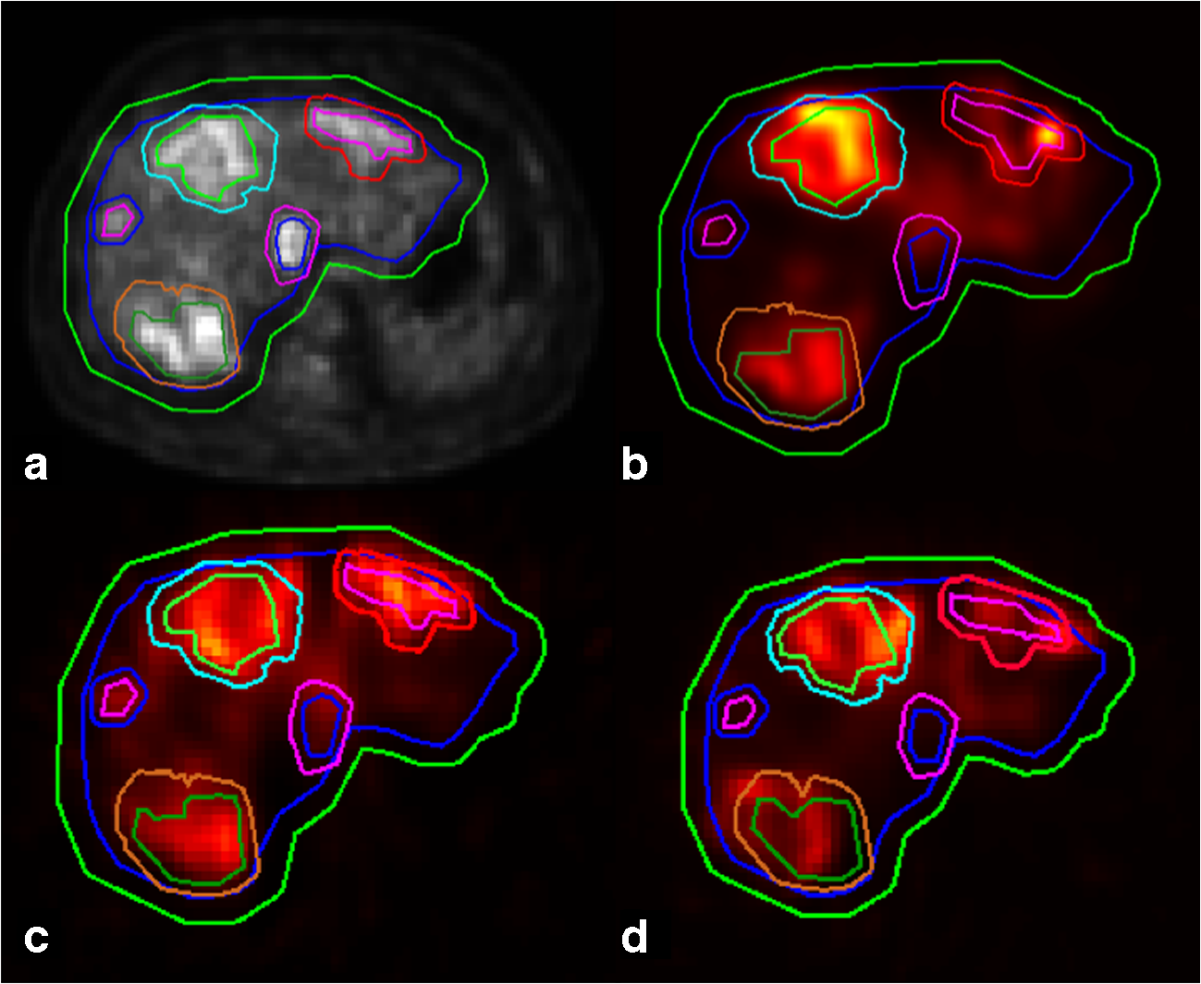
Example of liver and tumor segmentation on FDG-PET (a) and co-registration on ^99m^Tc-MAA (b), ^166^Ho-scout (c), and ^166^Ho-therapeutic dose (d) SPECT images. The delineated VOIs were extended with a margin of 1 cm; therefore, each target volume is surrounded by a second line
